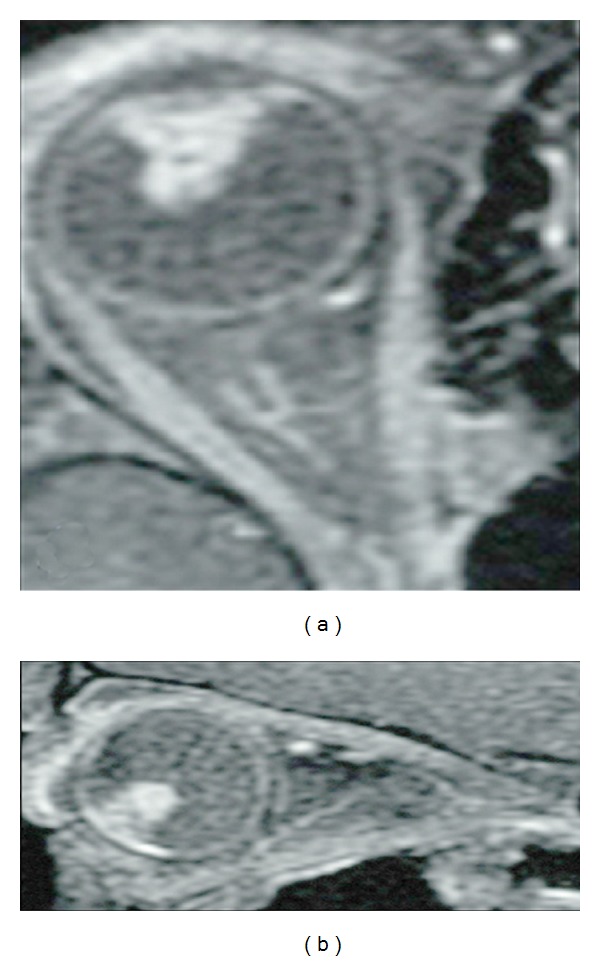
Axial (a) and sagittal (b) T1-weighted postcontrast images with fat saturation show an irregular, enhancing mass arising from the nonpigmented epithelium of the ciliary body. The tumor is confined to the globe. *Case courtesy of Dr. M. Mafee, UCSD Department of Radiology*.
